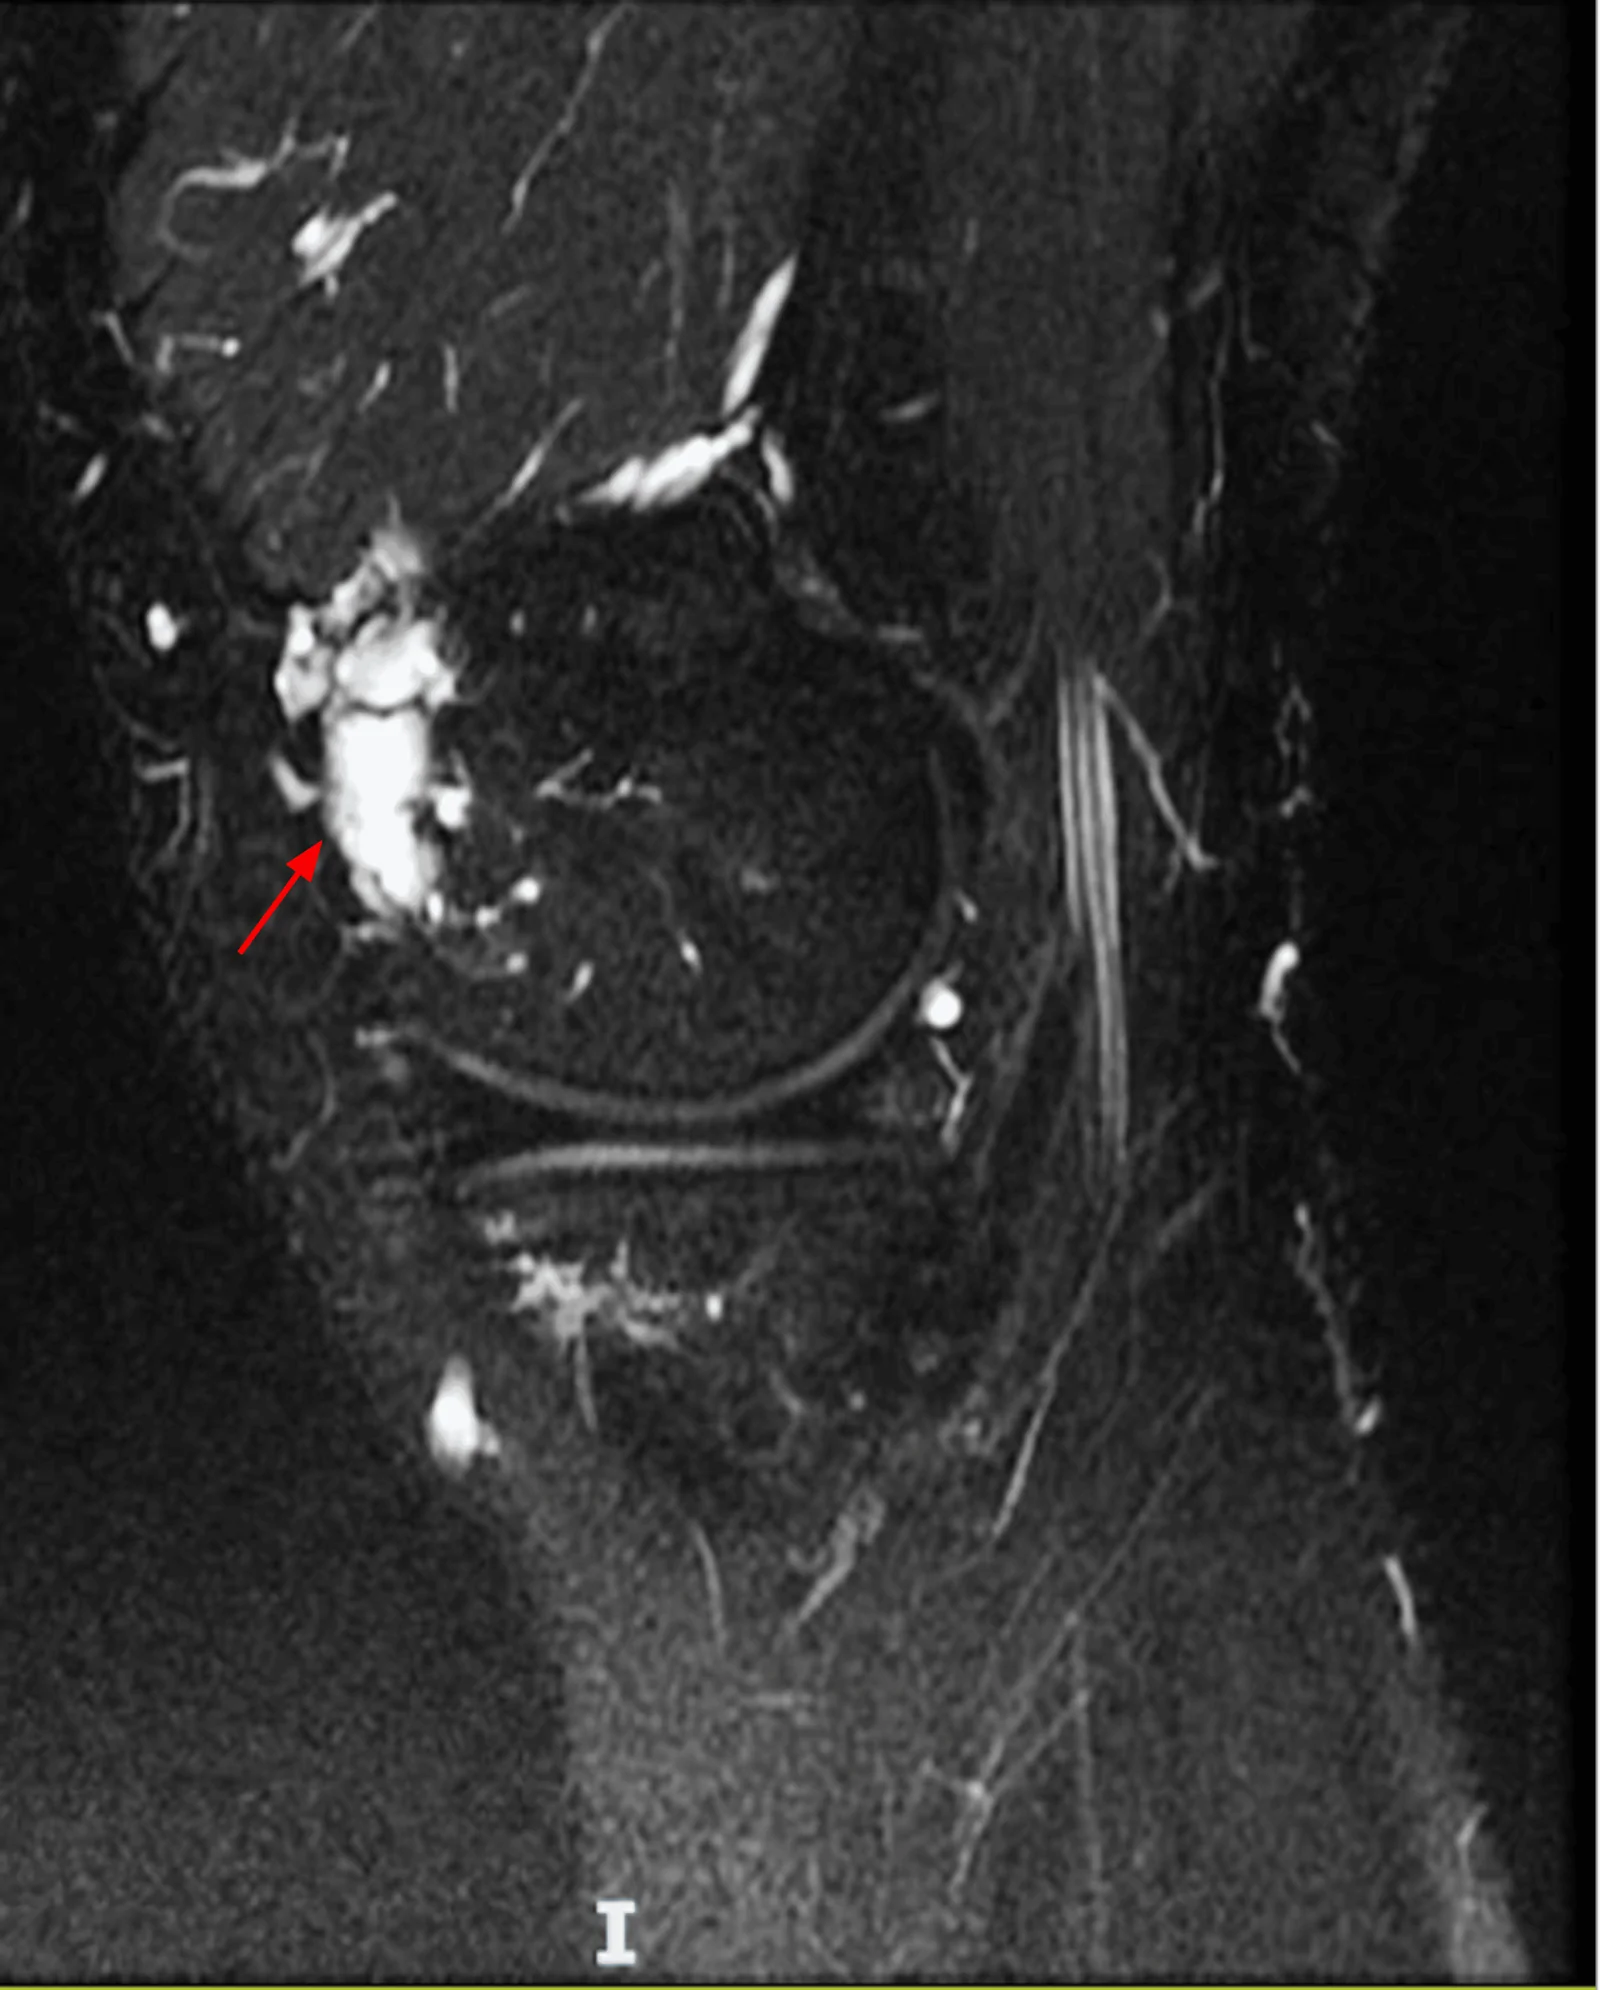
MRI of the right knee demonstrating a slow-flow vascular malformation.
